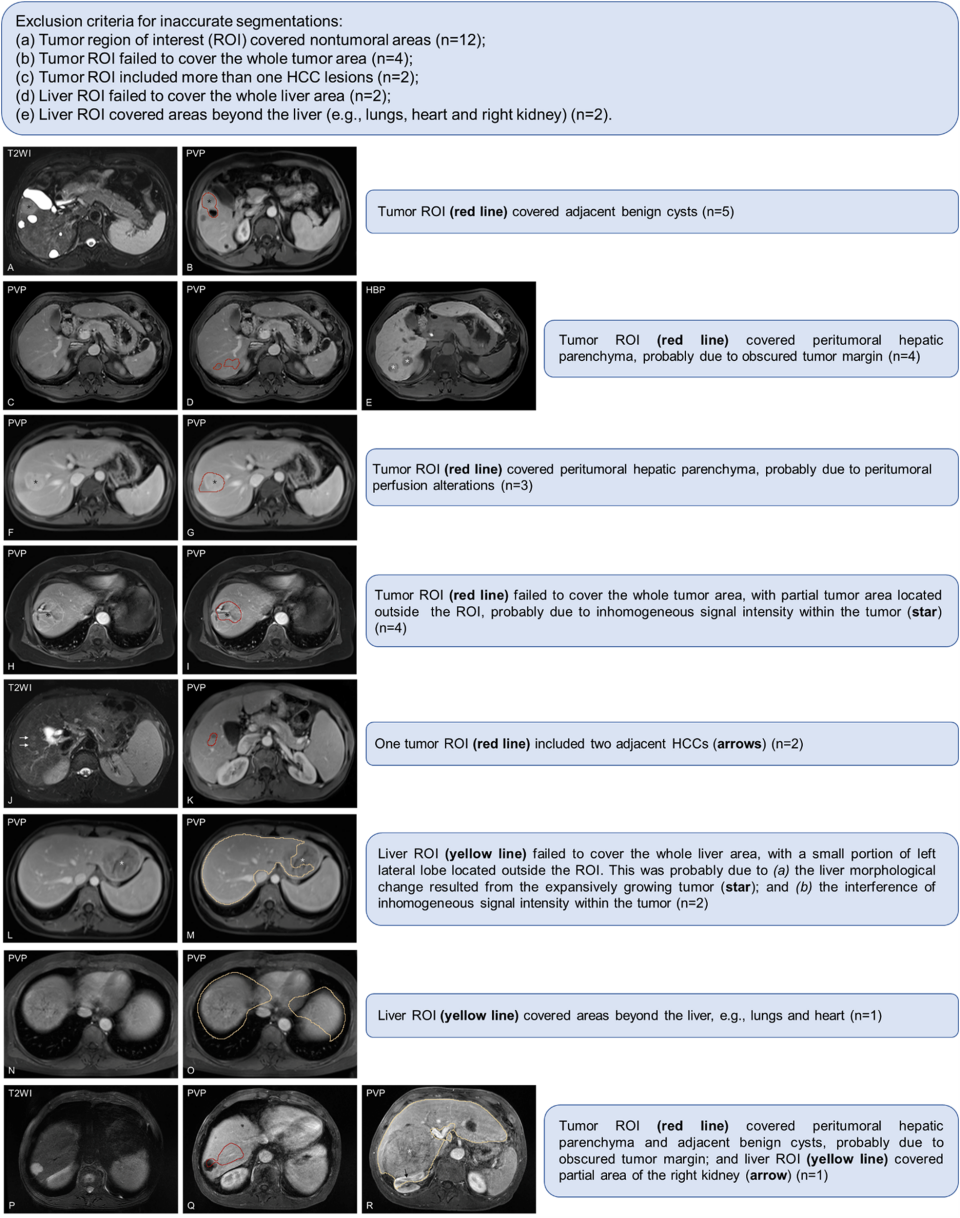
A–R Examples of inaccurate image segmentations. HBP, hepatobiliary phase; HCC, hepatocellular carcinoma; PVP, portal-venous phase; ROI, region of interest; T2WI, T2-weighted imaging
